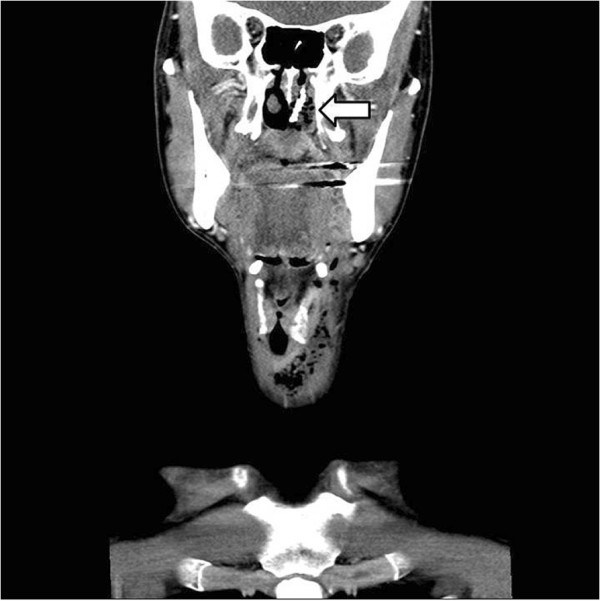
Coronal view of same CT. (Arrow) A neck CT shows the toothbrush spanning the nasopharynx and oropharynx, as well as subcutaneous emphysema in the anterior and lateral cervical regions.
